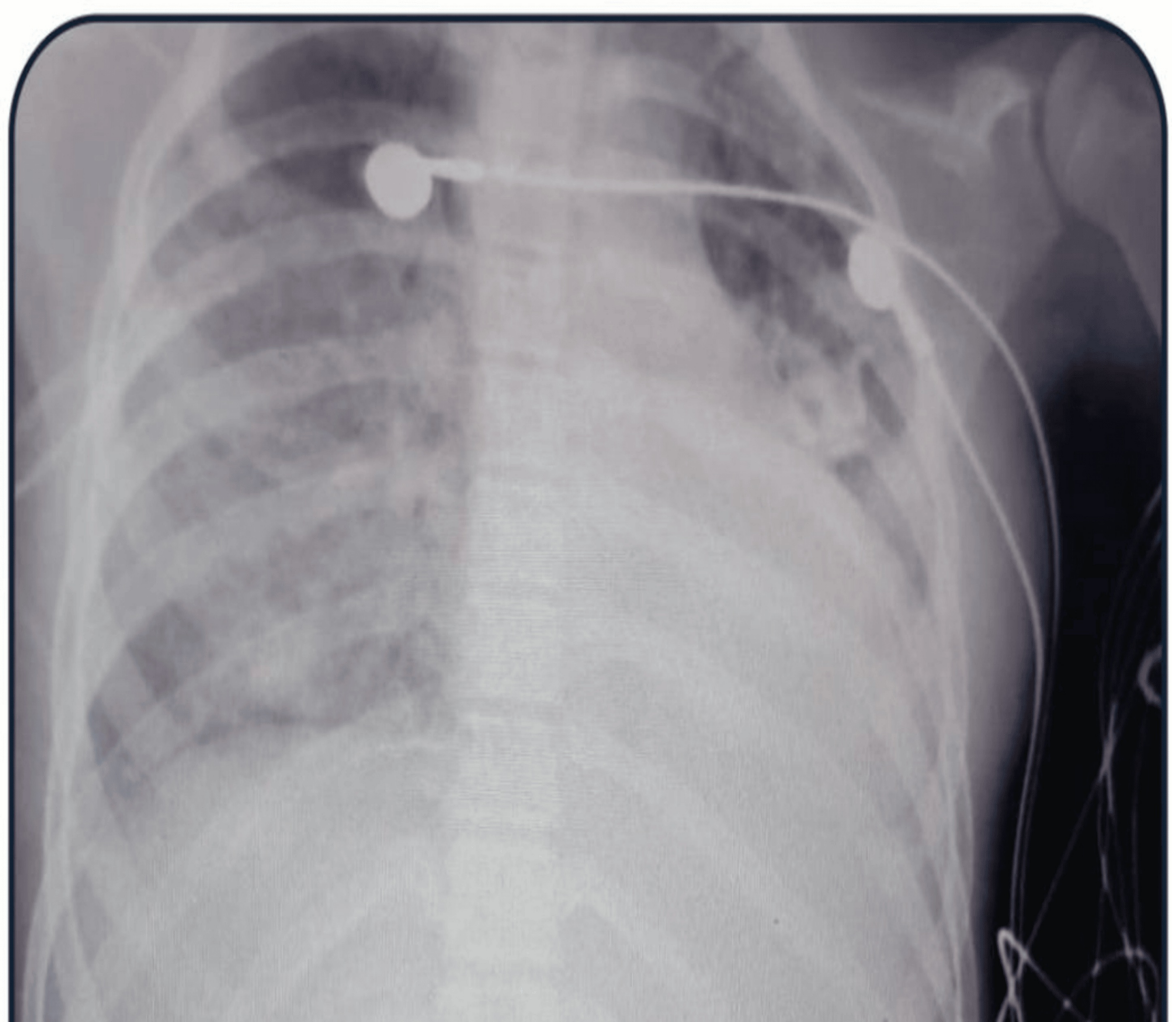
Chest radiograph showing generalized bilateral lung infiltrates
